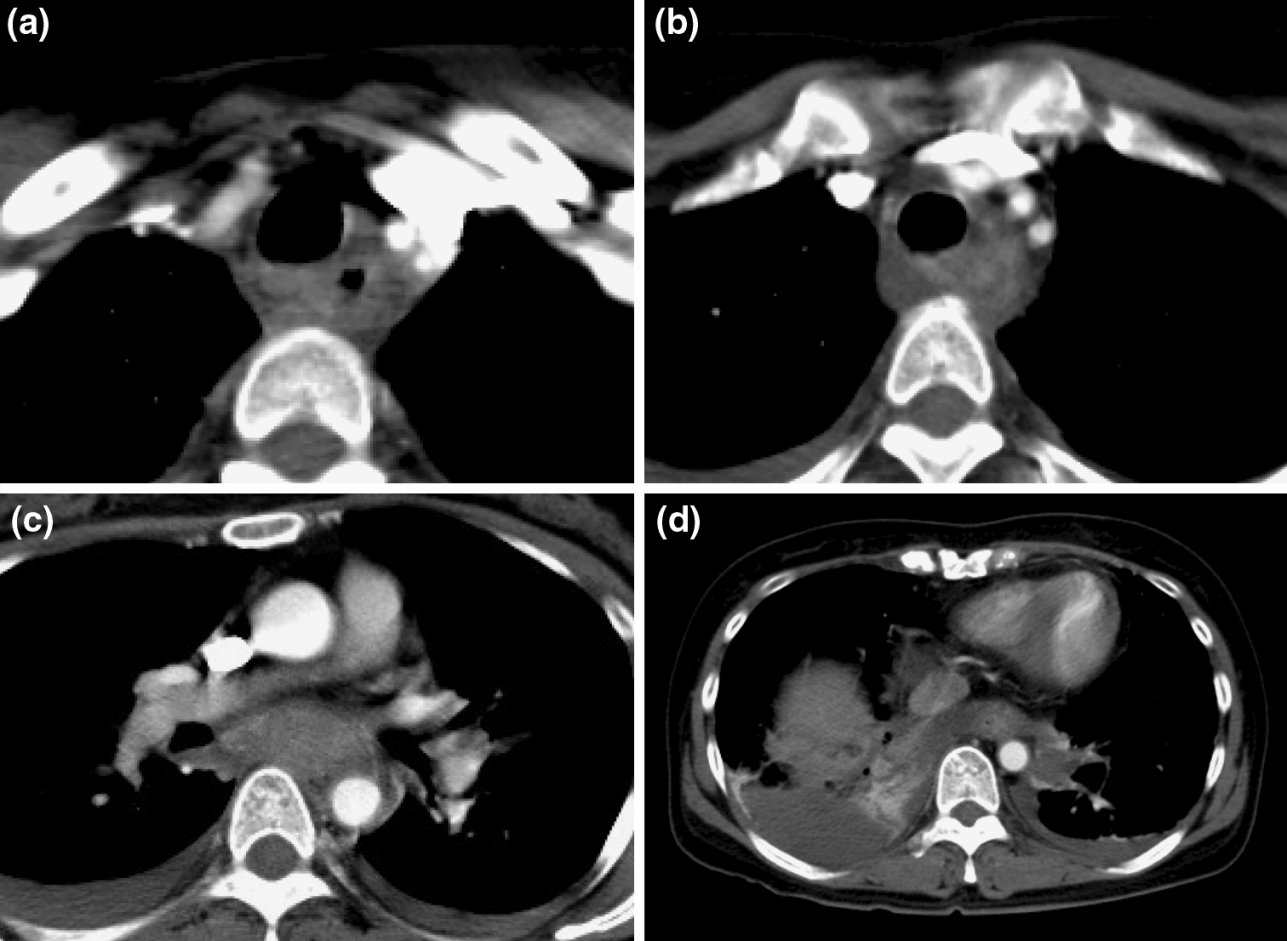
An enhanced computed tomography (CT) scan conducted on the day of onset showing diffuse thickening of the esophagus and edematous enlargement of the posterior mediastinum with bilateral pleural effusions. Sections are shown at the level of the cervical esophagus (a), the thoracic inlet (b), the mid-esophagus (c), and the lower esophagus (d). Note the low attenuation surrounded by a peripheral enhancing rim (b)
